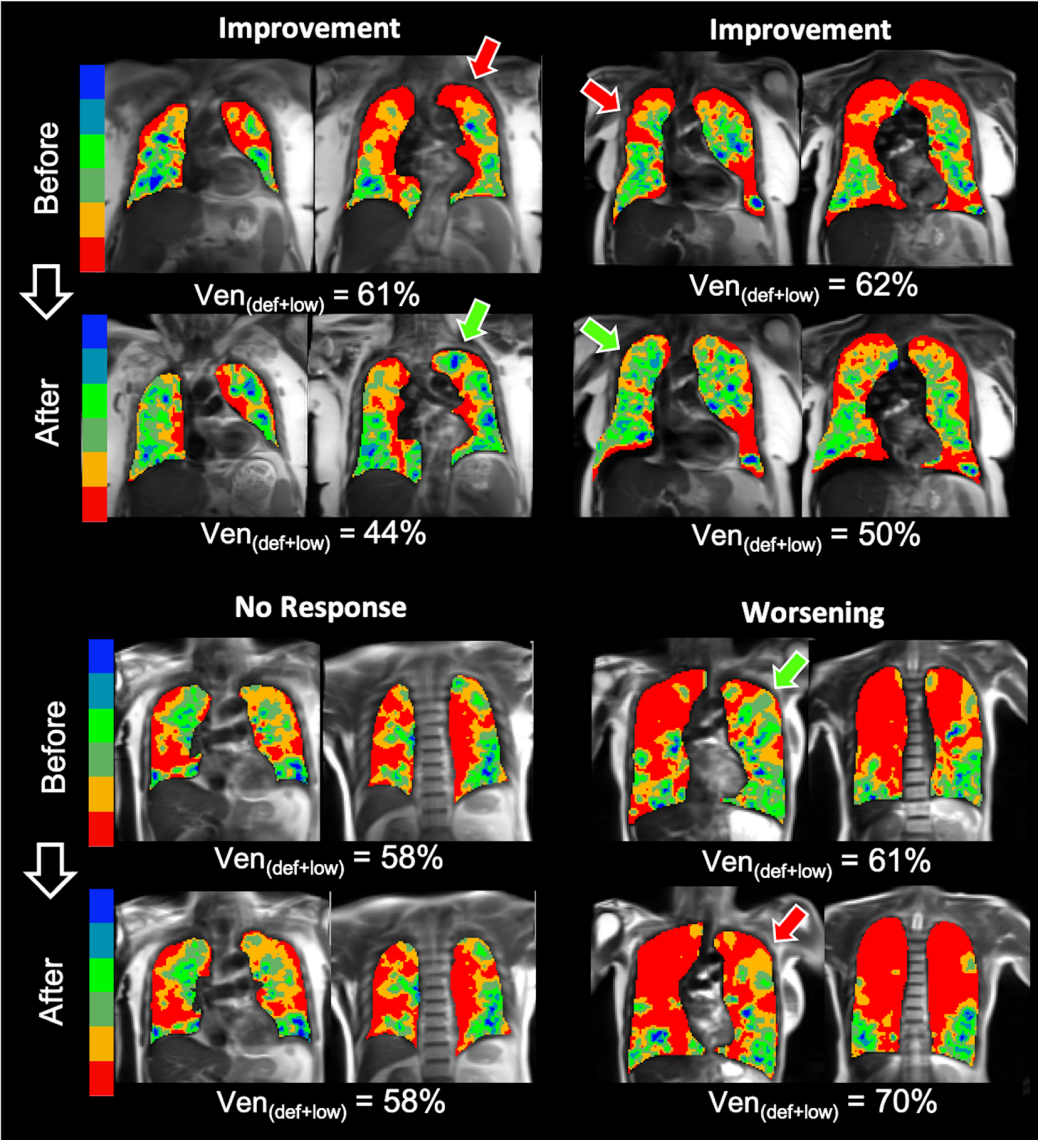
^129^Xe magnetic resonance imaging (MRI) ventilation maps before and after therapy from four representative subjects. The subjects in the top row showed improved (i.e., reduced) ven_def + low_ following therapy, while subjects in the bottom row exhibited no change (left) or worsening (right). Arrows indicate prominent regions where ventilation changed following therapy.
